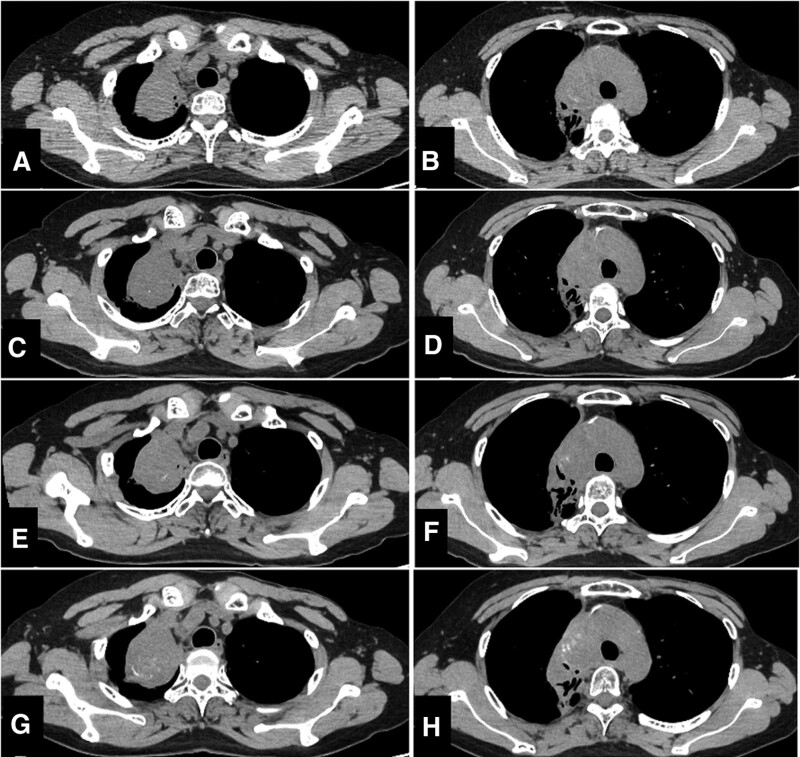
Computed tomography of the tumor during larotrectinib therapy. Larotrectinib treatment began at 200 mg orally daily; no dose reduction has been needed. The CT showed stable disease at 2 mo (A, B), 5 mo (C, D), 11 mo (E, F), and 21 mo (G, H) from commencement of larotrectinib treatment. CT = computed tomography.
